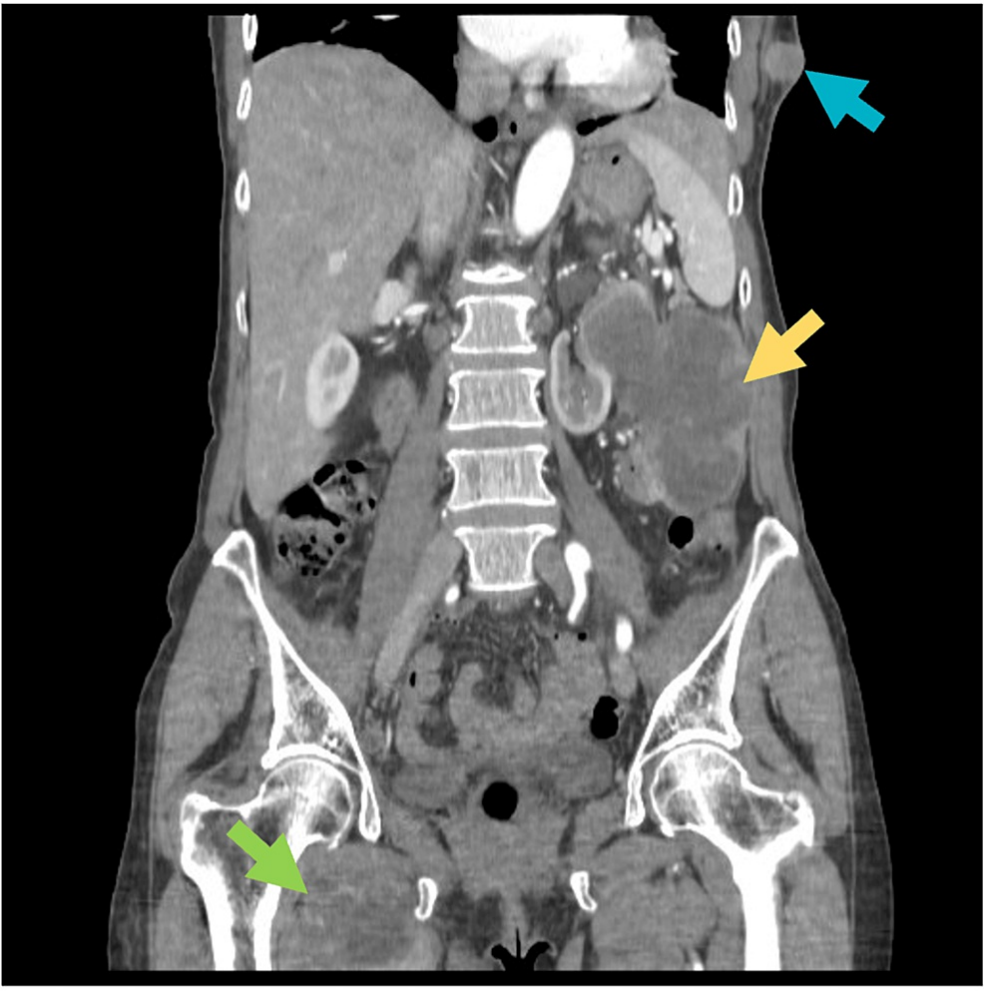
Masses on CT scan The blue arrow shows the left thoracic mass, the yellow arrow shows the left retroperitoneal mass, and the green arrow shows the inner right thigh mass.
